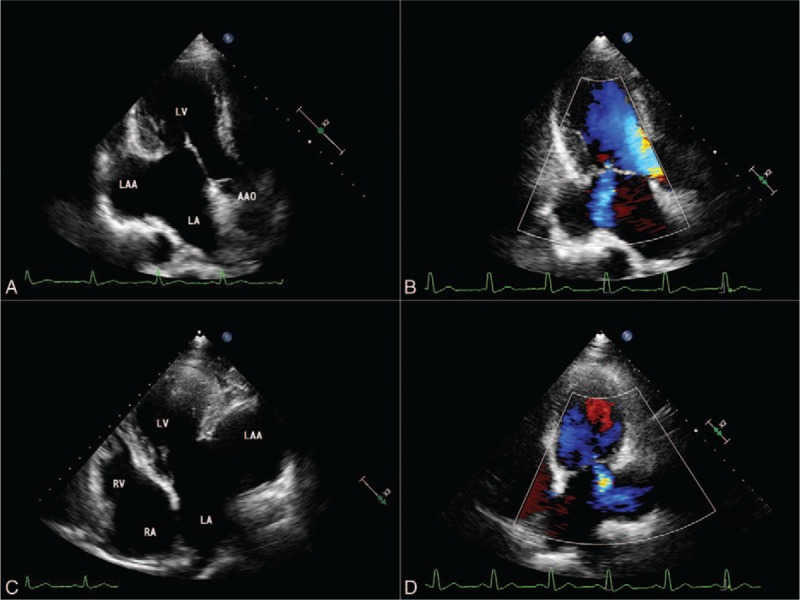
Transthoracic echocardiograms. Apical 3-chamber (A, B) and 4-chamber (C, D) views showing a 8.4 × 6.8 × 4.3-cm size mass communicating to LA and the presence of mild mitral regurgitation. AAO = ascending aorta, LA = left atrium, LAA = left atrial appendage, LV = left ventricle, RA = right atrium, RV = right ventricle.
